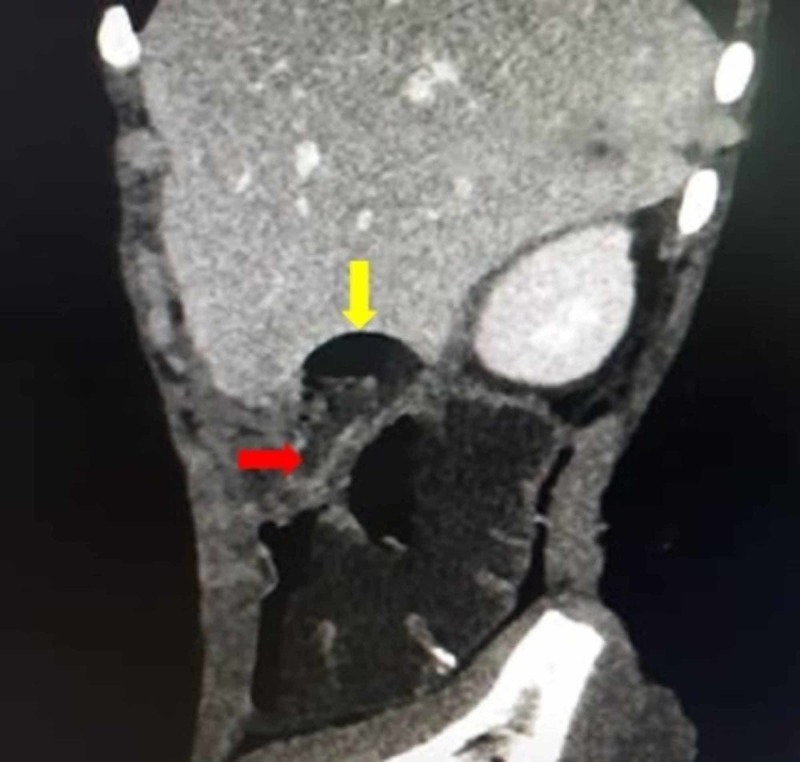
Sagittal CT scan of the abdomen depicting a dilated inflamed appendix with a perforated tip and an air pocket. The yellow arrow shows the perforated tip of the appendix with collection. The red arrow shows the base of the appendix.
